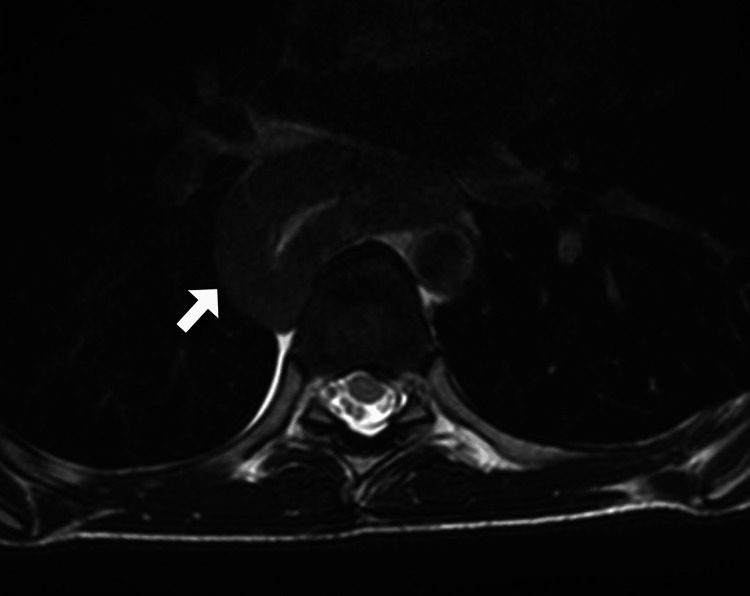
Axial T2-weighted MR image of the chest revealing diffuse circumferential wall thickening of the esophagus (arrow). MR: magnetic resonance
